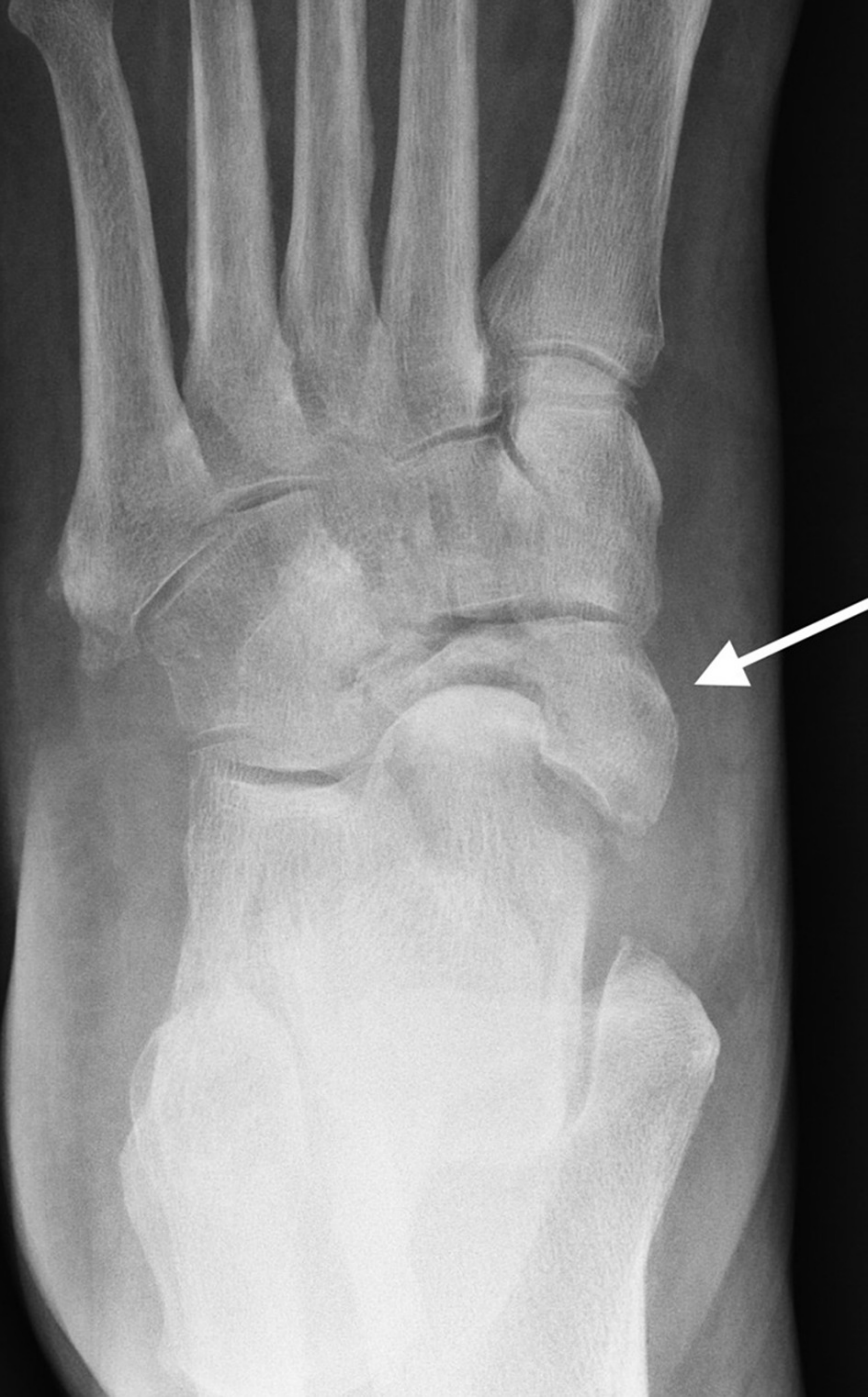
Radiograph of the left foot of a 63-year-old female demonstrates isolated destruction of the navicular (arrow) with remaining tarsal bones demonstrated to be intact. Features are consistent with established navicular osteonecrosis (Mueller–Weiss syndrome).
